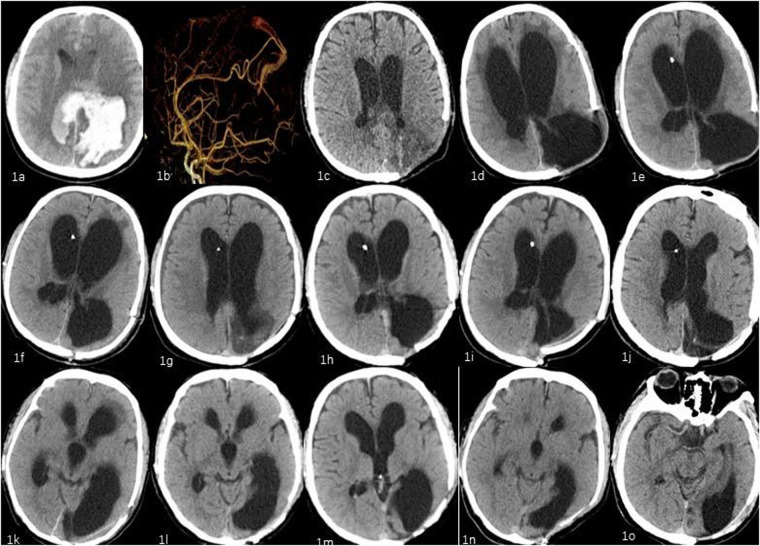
Serial imaging findings throughout the patient's clinical course (a) axial cranial CT demonstrating a large hyperdense hematoma involving the splenium of the corpus callosum and left parieto-occipital lobe.(b) Axial cranial CTA revealing a left parieto-occipital arteriovenous malformation with dilated feeding arteries and tortuous draining veins.(c) Postoperative axial CT following emergency craniotomy for hematoma evacuation, AVM resection, and decompressive craniectomy.(d) Axial CT showing communicating hydrocephalus at 1 month after the index surgery.(e) Postoperative axial CT after VP shunt placement demonstrating resolution of hydrocephalus.(f,k) Postoperative axial and coronal CT images at 1 month post-VP shunt placement showing autologous cranioplasty for the left parieto-occipital skull defect.(g,l) Axial and coronal CT images at 3 months post-cranioplasty showing stable bone flap position with no resorption.(h,m) Axial and coronal CT images at 6 months post-cranioplasty showing mild focal resorption and thinning of the bone flap.(i,n) Axial and coronal CT images at 1 year post-cranioplasty showing significant bone resorption with focal loosening and decompression window depression.(j,o) Axial and coronal CT images at 3 years post-cranioplasty showing progressive bone resorption with regional variability. All images are submitted as a 3 × 5 grid at 300 DPI resolution with enlarged subfigure labels (a–o) for optimal readability.
